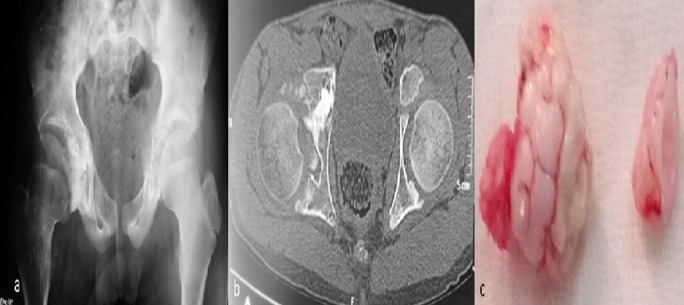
(A) pelvic X-ray, widening of the hip joint space with organized calcifications of the roof of the acetabulum; (B) CT scan showed many intra-acetabular osteochondroma; (C) macroscopic aspect of intraarticular chondroma
